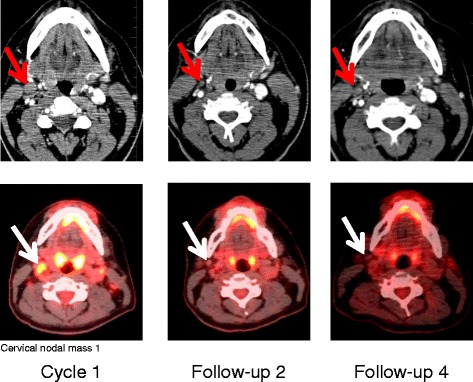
Positron emission tomography-computed tomography scans showing response to single-agent MOR208 therapy (arrow)
